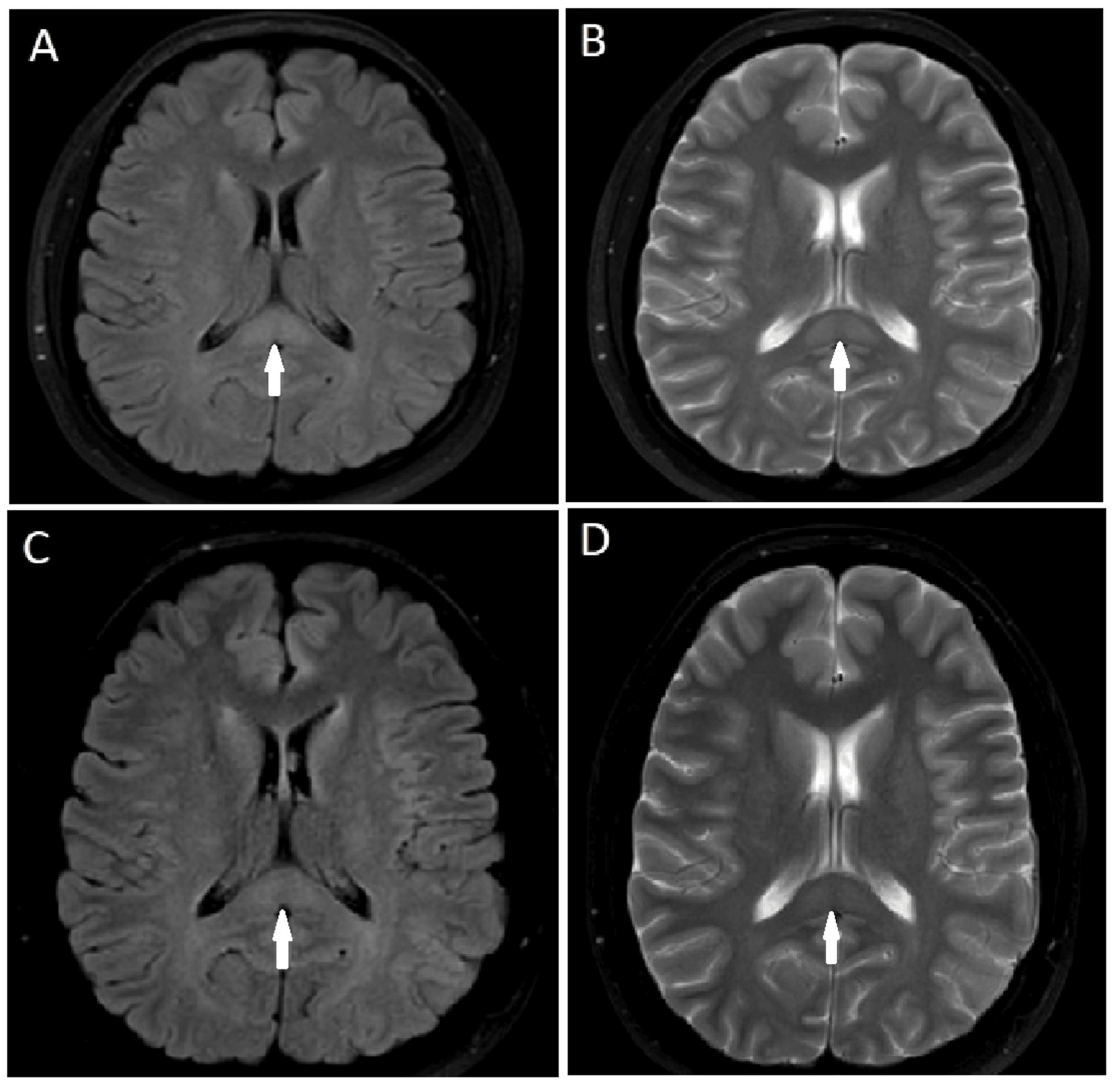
Follow-up brain MRI results. Axial FLAIR (A) and T2-weighted (B) MRI at six weeks demonstrate partial resolution of the lesion (white arrows). Follow-up brain MRI at six months shows near-complete resolution of the lesion in axial FLAIR (A) and T2-weighted (B) images (white arrows). MRI: magnetic resonance imaging; FLAIR: fluid-attenuated inversion recovery
